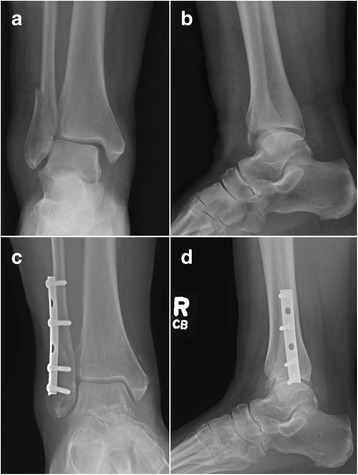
Case example of a 65 year-old female patient who sustained an unstable SER-4 equivalent right ankle fracture (a, b). The injury was managed by open reduction with internal fixation of the lateral malleolar fracture using a posterolateral antiglide plate and four pretapped Bone-Screw-Fasteners. The fracture healed uneventfully in anatomic position, as demonstrated on 6 months follow-up radiographs (c, d)
